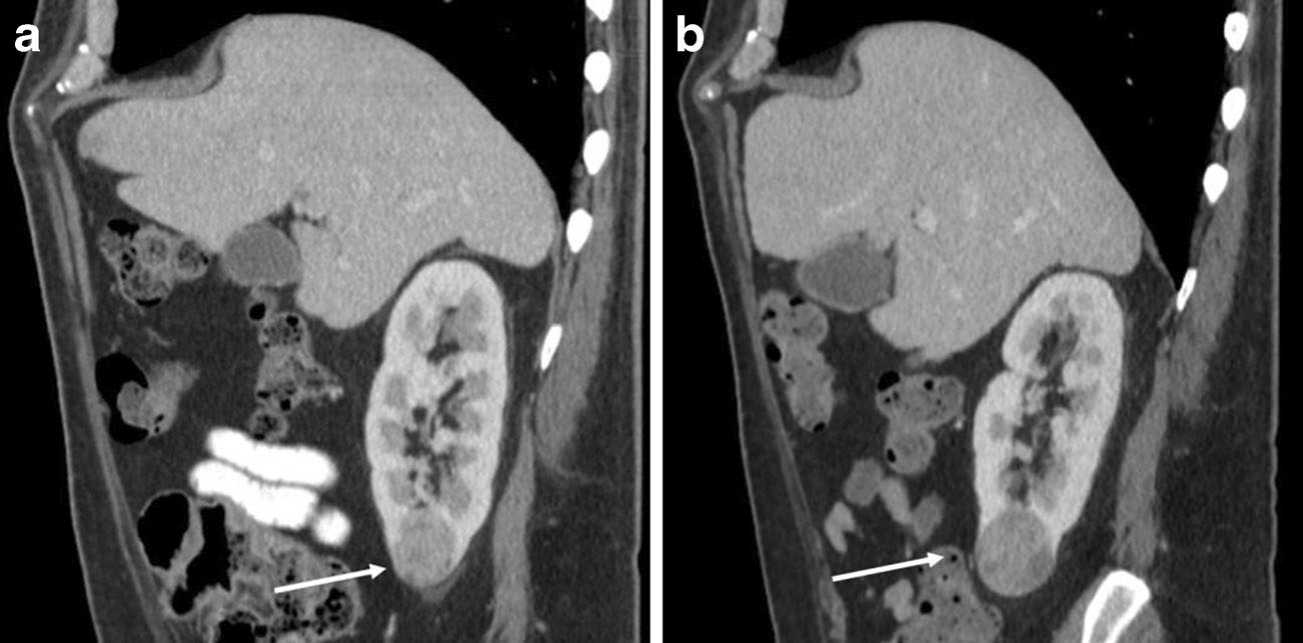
A 61-year-old man with a right renal oncocytoma demonstrating interval growth. a The sagittal reformatted image in the portal venous phase of a CE-CT demonstrates a moderately enhancing well-circumscribed mass in the lower right renal pole (*arrow*). The mass measures 3.5 cm in long axis diameter. b Follow-up CT 2 years after a demonstrates interval increased size of the mass (*arrow*), now measuring 4 cm
